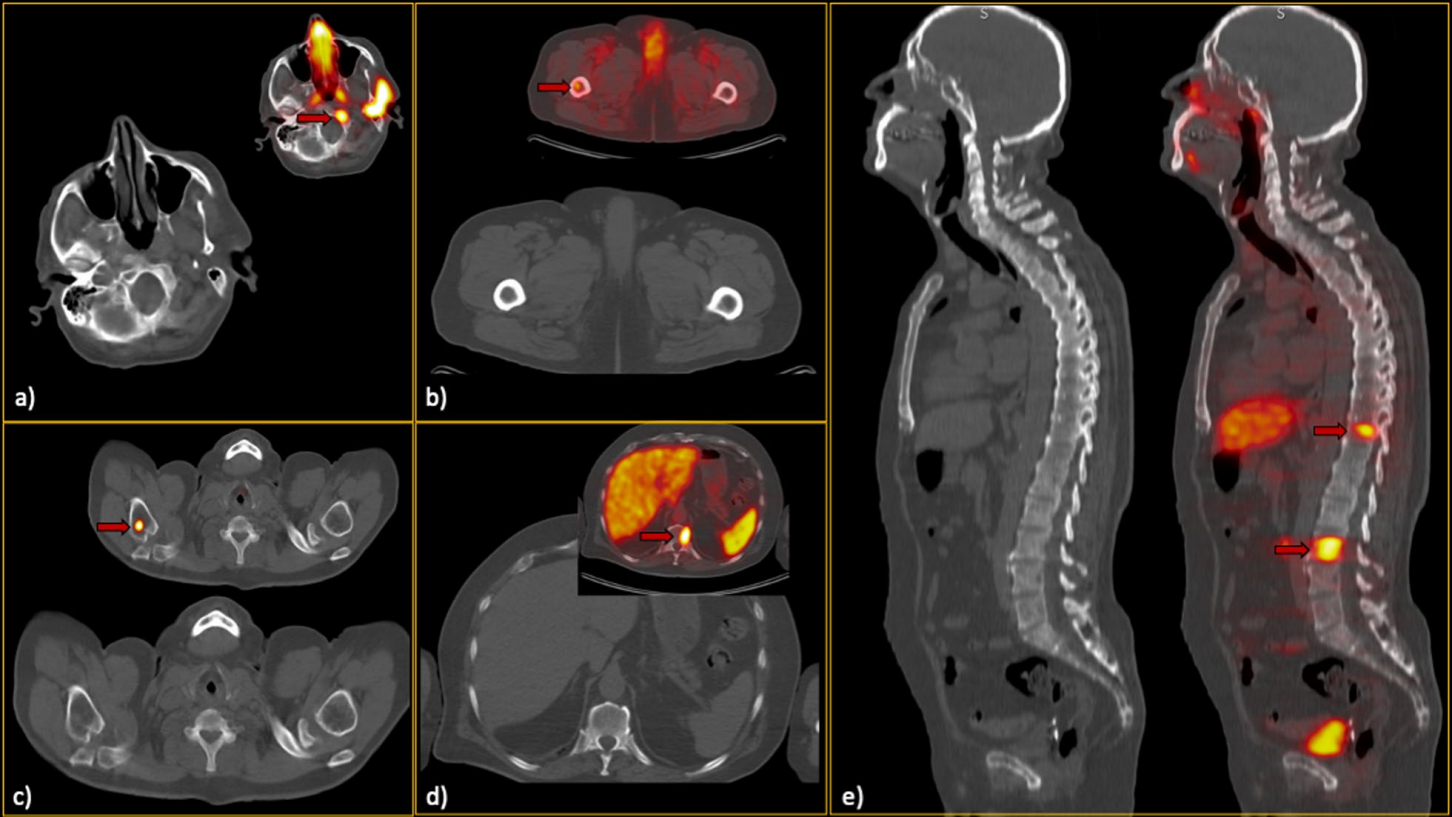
False-negative low-dose CT findings (red arrows). In each section (a–e), the CT-alone images, along with the hybrid ^68^Ga-PSMA PET/CT images, can be found. Regarding section a), it should be noted that he was one of our interesting cases, as he showed only a single bone metastasis in the skull base. Thus, although the sclerotic lesion can be easily seen in this single slice, it was not prominent enough while scrolling, since the remainder of the skeleton was free of metastases. Furthermore, in section c), subtle sclerosis could be found retrospectively; however, it was not considered a significant lesion by all readers when reviewing CT images. Notably, this false finding did not misclassify the patient’s disease burden eventually
